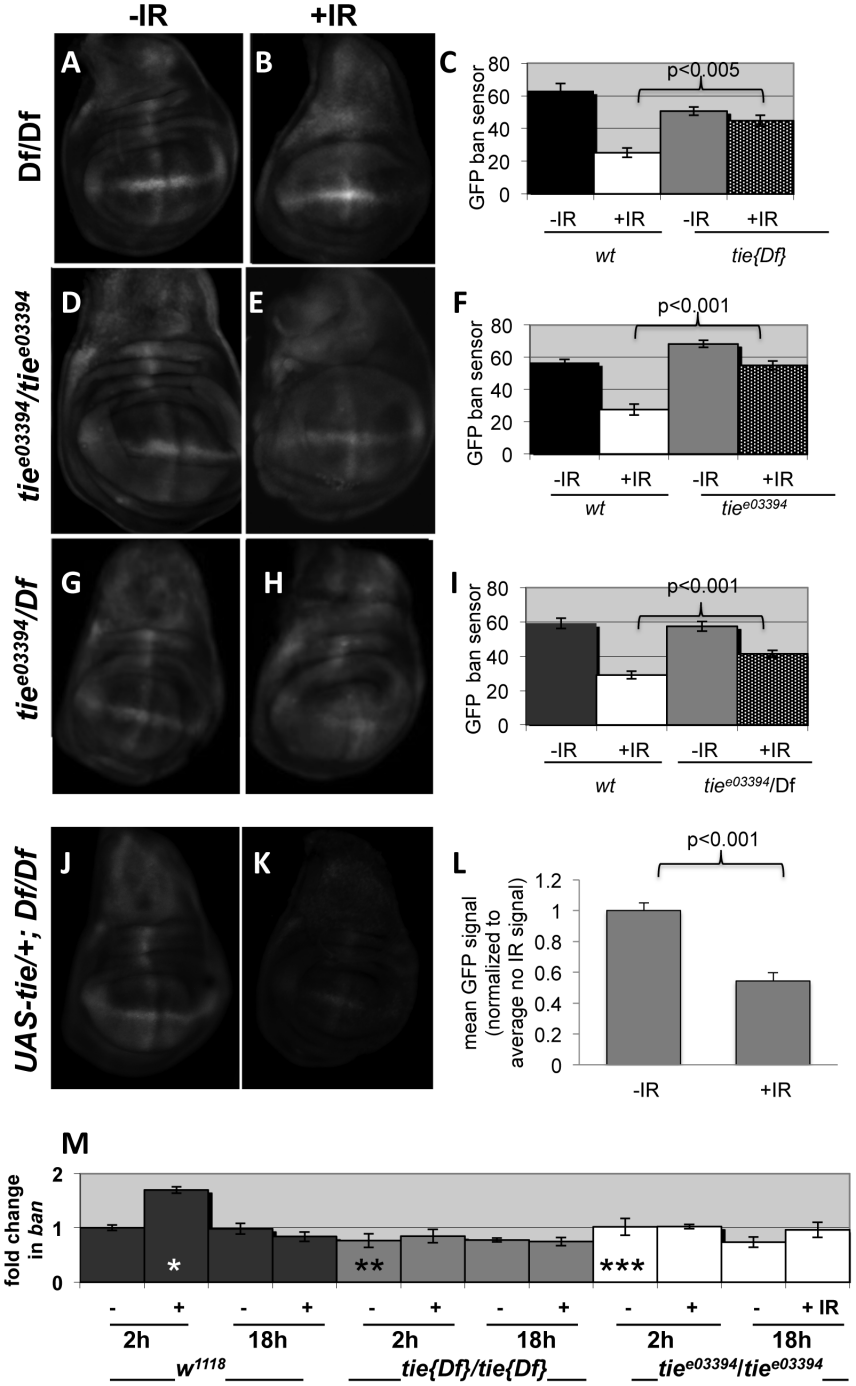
tie* is needed for IR-induced changes in GFP *ban* sensor. (A–I) Third instar larvae were irradiated at 96±2 hr after egg deposition with 0R (-IR) or 4000R (+IR) of X-rays and wing discs imaged live 24 h later. Images were acquired and treated identically. The mean GFP signal was quantified for each disc using Image J, and the averages are shown for each genotype/treatment. Age-matched sensor only controls (i.e. wild type for *tie*) were included in each experiment (images not shown here but see Fig. S1). (A–C) Wing discs from homozygotes for Df(3L)Exel2098 that removes only *tie* (‘*tie*
